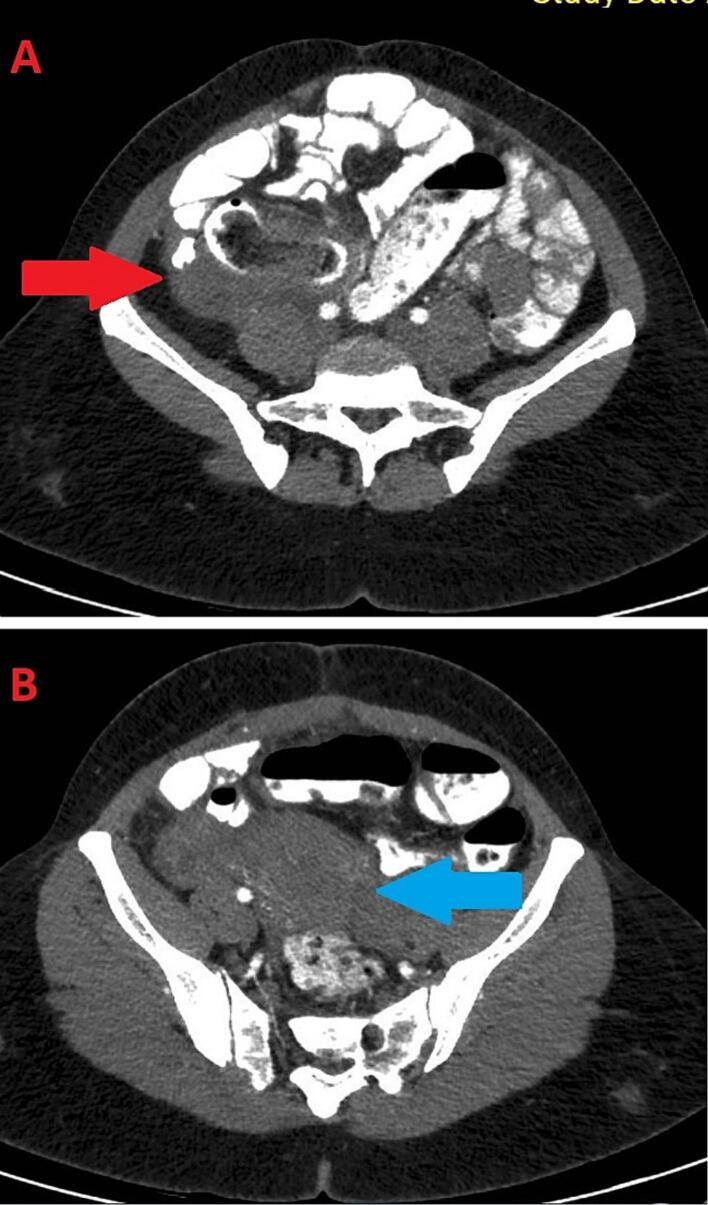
CT scan of abdomen with oral and intravenous contrast axial view. A – Showing illdefined iflamatory mass in right illiac fossa involving ileoceacal region with hazziness and streaking with poor plane of demarcation(red arrow). B – Showing uterus being pulled towards the right illiac inflamatory mass (blue arrow).
